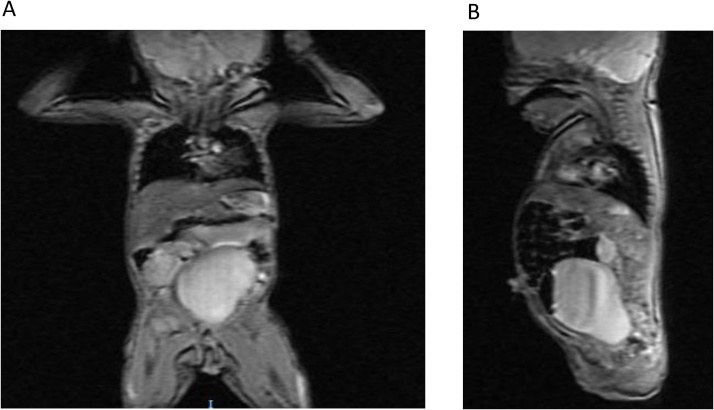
AP and lateral MRI views of infant showing massive hydrocolpos. A: AP MRI view of the infant showing hydrocolpos measuring 4.6 × 5.0 × 5.7 cm and causing severe mass effect on abdominal structures. B: lateral MRI view of the infant showing hydrocolpos causing severe mass effect on abdominal structures.
